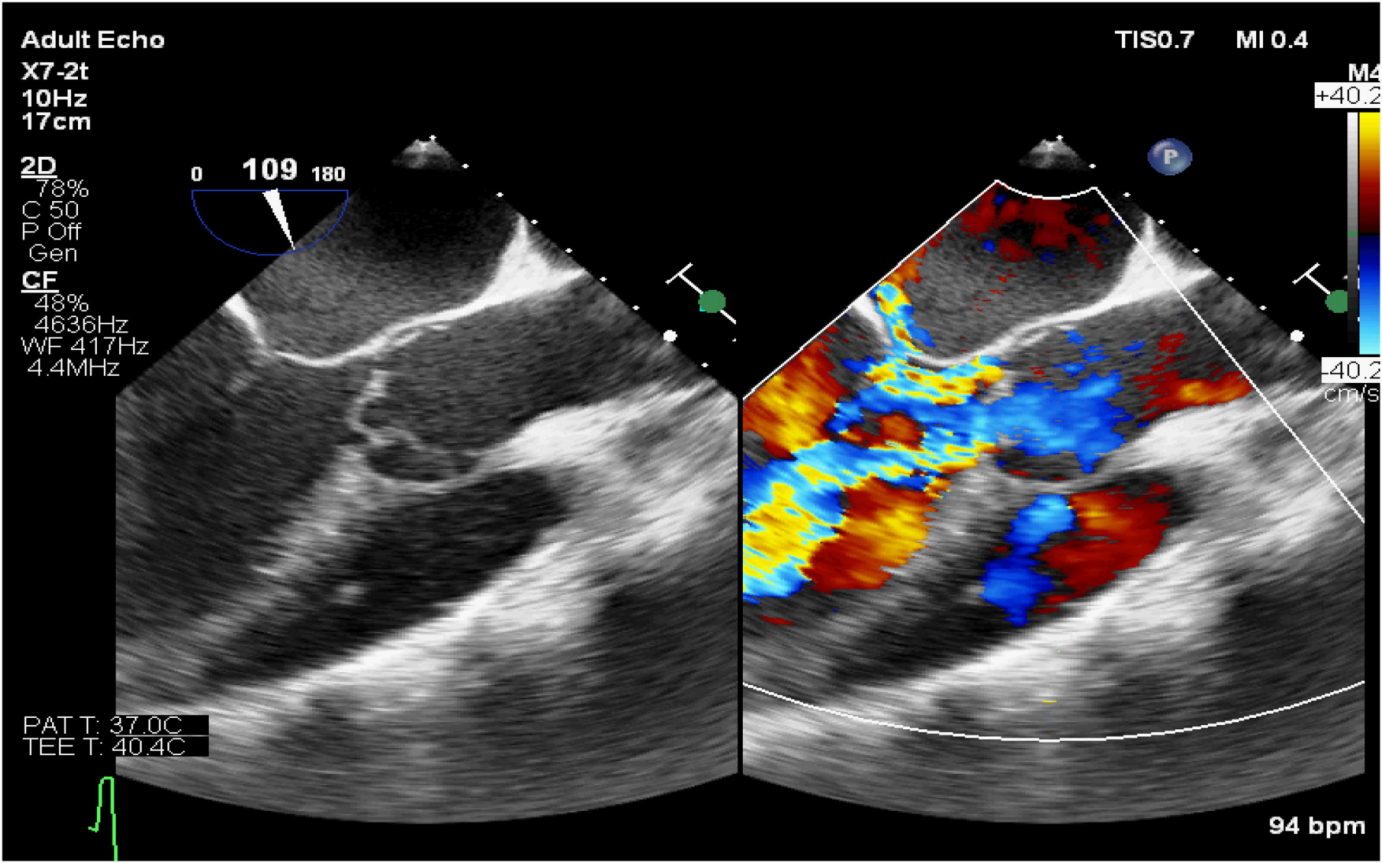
Transesophageal echocardiography (long-axis view of the left ventricle). Left panel: Rupture and detachment of the left and right coronary valve annuli, with the valve leaflets swinging along with the annuli. Right panel: Severe aortic valve regurgitation is observed.
